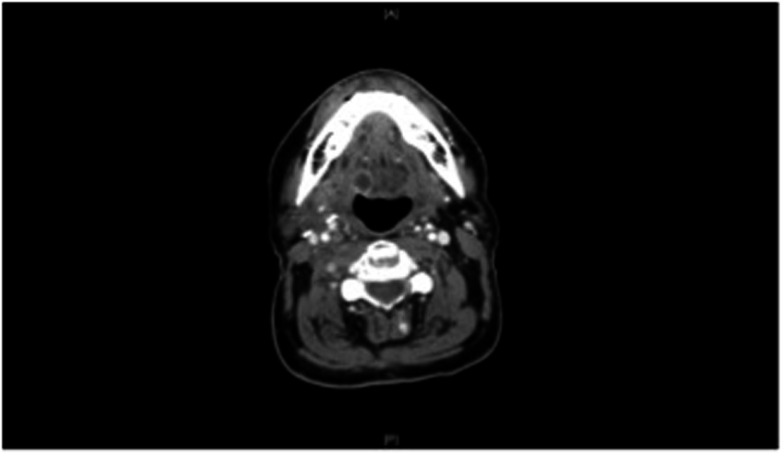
CT neck of the neck with contrast, prior to treatment, demonstrates a submucosal irregularity that was ultimately biopsied and diagnosed as recurrent squamous cell carcinoma.
